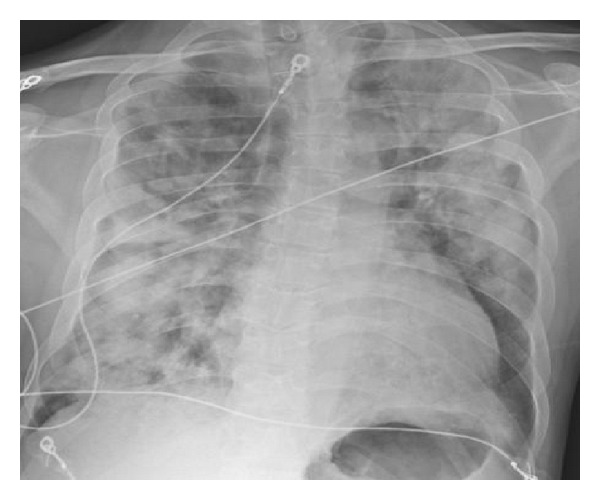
Chest X-ray showing diffuse bilateral patchy infiltrates at admission.
